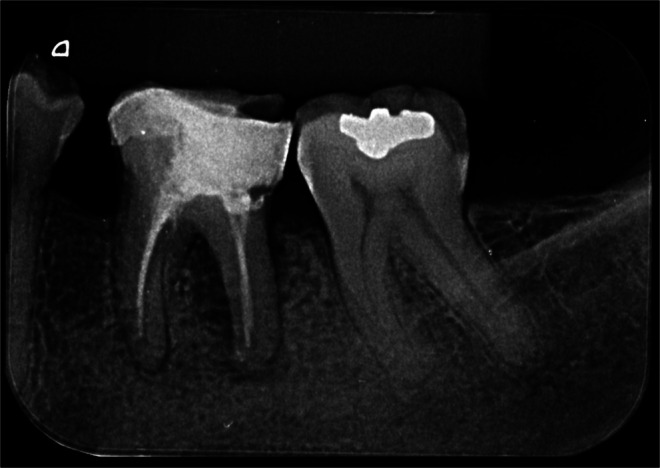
Periapical radiograph (PR) of a left mandibular first molar with previous root canal treatment, showing periradicular radiolucency—interpretation stage 1.
